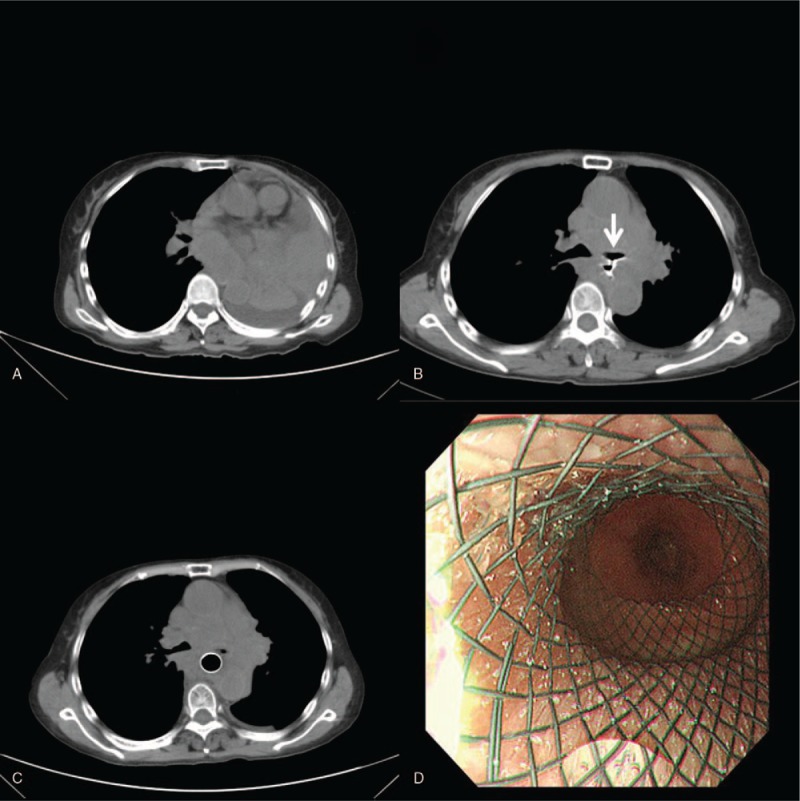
Chest computed tomography (CT) scan showed mediastinal and left hilar mass, along with left total lung atelectasis (A). Left total lung atelectasis disappeared, however, acquired esophago-tracheobronchial fistula (thin arrow) occurred after anlotinib treatment (B). A fully covered self-expandable metallic stent was placed immediately after the diagnosis (C,D).
